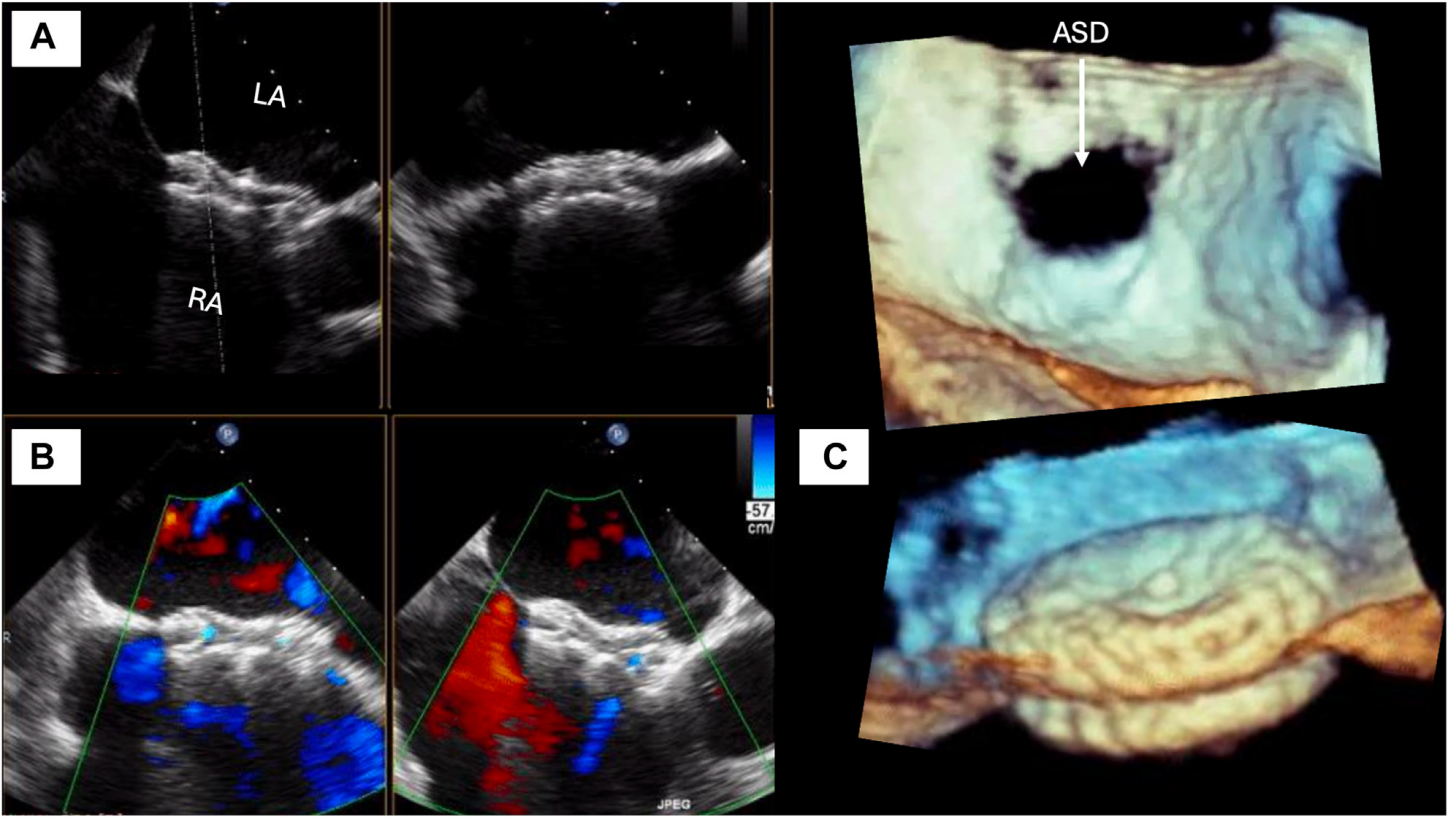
Two-dimensional transesophageal echocardiography, orthogonal biplane atrial septal views without (A) and with (B) color-flow Doppler, demonstrates the normal appearing, well-positioned septal occluder device without residual shunting. Three-dimensional transesophageal echocardiography, volume-rendered display of the ostium secundum ASD (C) from the perspective of the RA at baseline (*top*) and a zoomed, oblique view after repair with a well-positioned septal occluder device (*bottom*). *LA*, Left atrium; *RA*, right atrium.
